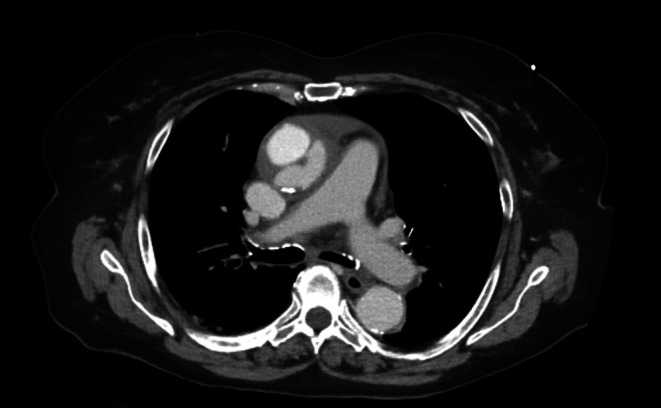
Axial computed tomography displaying Stanford type A/DeBakey type II acute aortic dissection.
